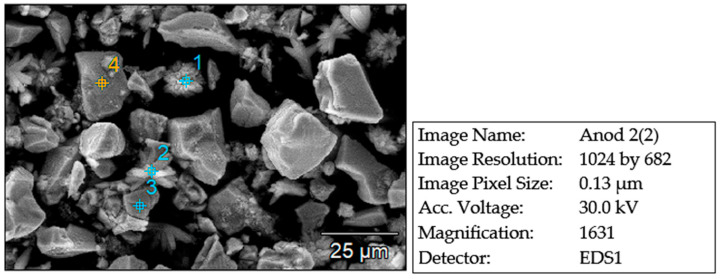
The morphology of the anodic paste recovered by ultrasonication in water.
